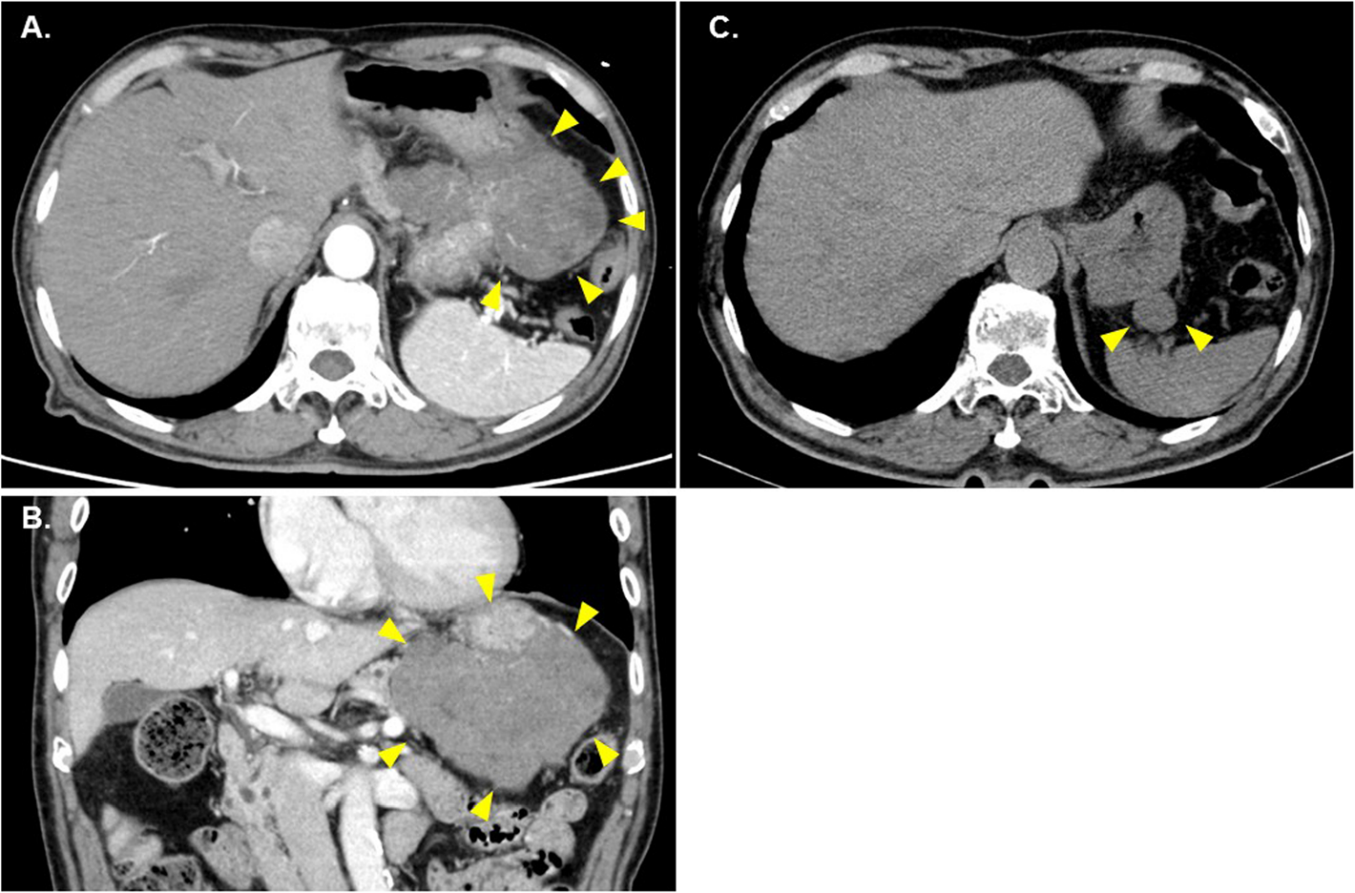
CT scan of the abdomen. a and b; at the time of admission. c; one and a half years ago of admission
